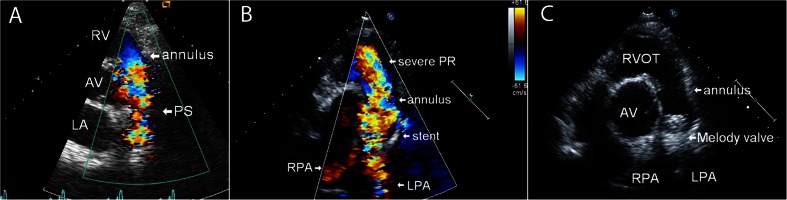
a Transthoracic echocardiographic image (parasternal short axis view) of the right ventricular outflow tract (RVOT) showing turbulent color Doppler flow across a stenotic pulmonary valve. AV = aortic valve. b Parasternal short axis view of RVOT with an indwelling stent in the main pulmonary artery well above the level of the pulmonary valve annulus and color Doppler flow showing severe pulmonary valve regurgitation (PR). Note the regurgitant reverse color Doppler flow in the left pulmonary artery (LPA) and right pulmonary artery (RPA). c Parasternal short axis view of the RVOT showing a Melody valve successfully deployed in the MPA
